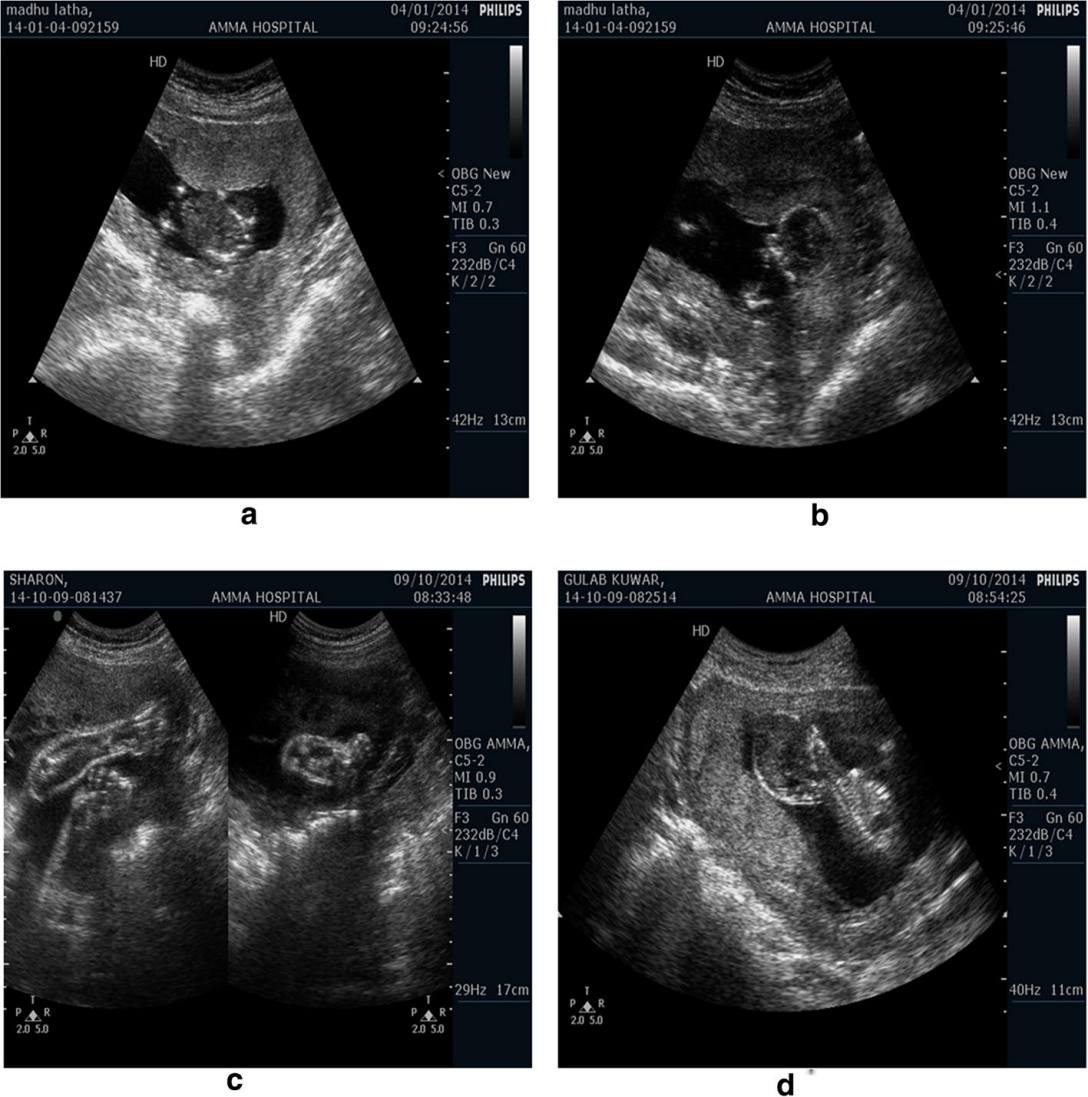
a–d Fetus Ultrasound Images captured at radiology lab of AMMA hospital of various patients
